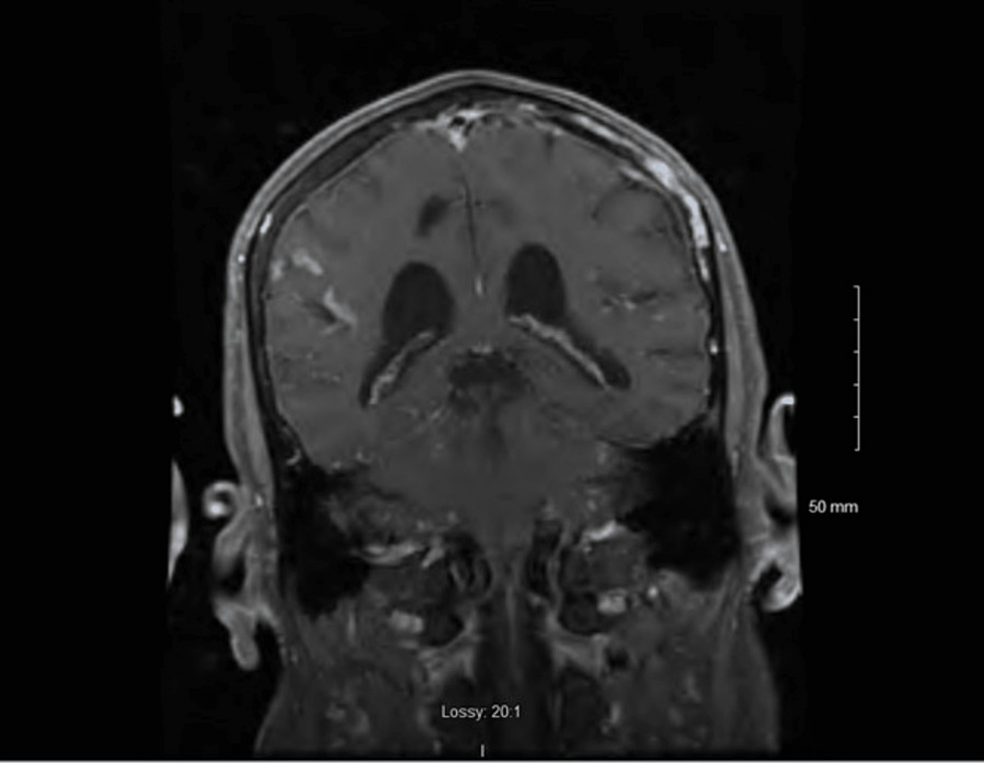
Coronal MRI showing temporal leptomeningeal enhancement
